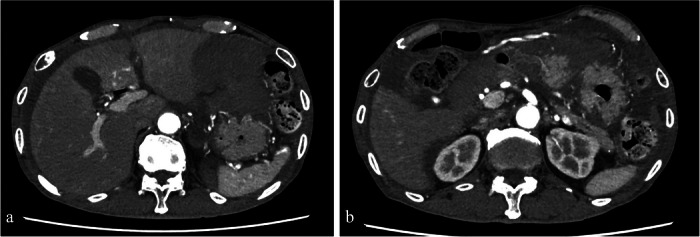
(a, b) Contrast‐enhanced computed tomography (CT) reveals a large hematoma around the gastrosplenic ligament and a localized hypoenhanced area in the posterior gastric wall. No contrast extravasation or vascular abnormality was identified on imaging.
